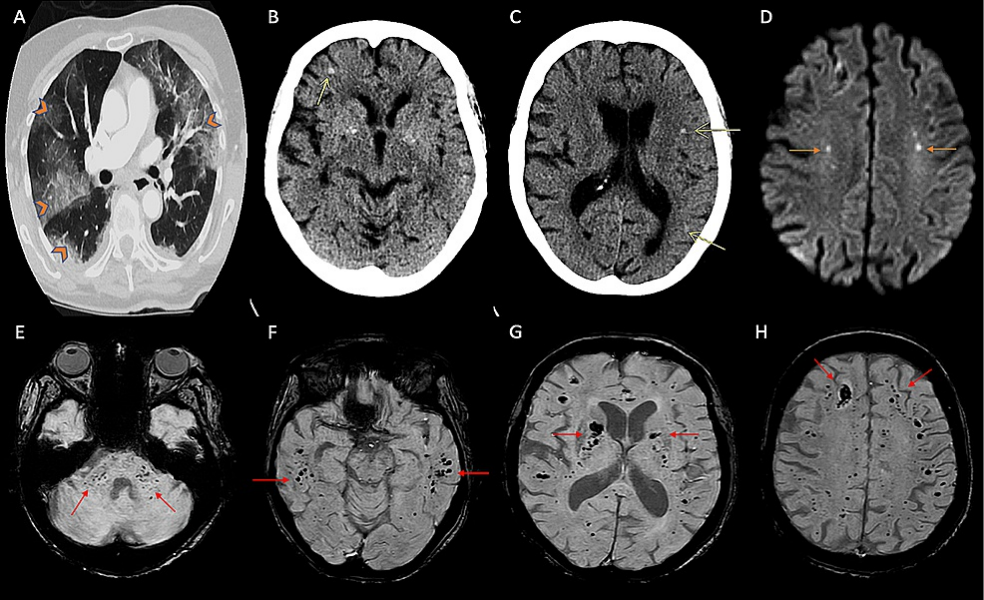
Imaging findings of patient 1 (A) CT (axial section) of the chest with contrast showing diffuse bilateral subpleural and perihilar ground-glass opacities (orange arrowheads). (B, C) CT head (axial sections) of the brain showing punctate hemorrhages in the right frontal and left frontal and parietal areas (yellow arrows). (D) MRI of the brain (axial section, diffusion-weighted imaging) showing hyperintensities in bilateral centrum semiovale areas (orange arrows). (E-H) MRI of the brain (susceptibility-weighted imaging) showing innumerable punctate microhemorrhages in the cerebellar peduncles and subcortical regions of bilateral hemispheres including bilateral basal ganglia and internal capsules (red arrows). CT, computed tomography; MRI, magnetic resonance imaging
